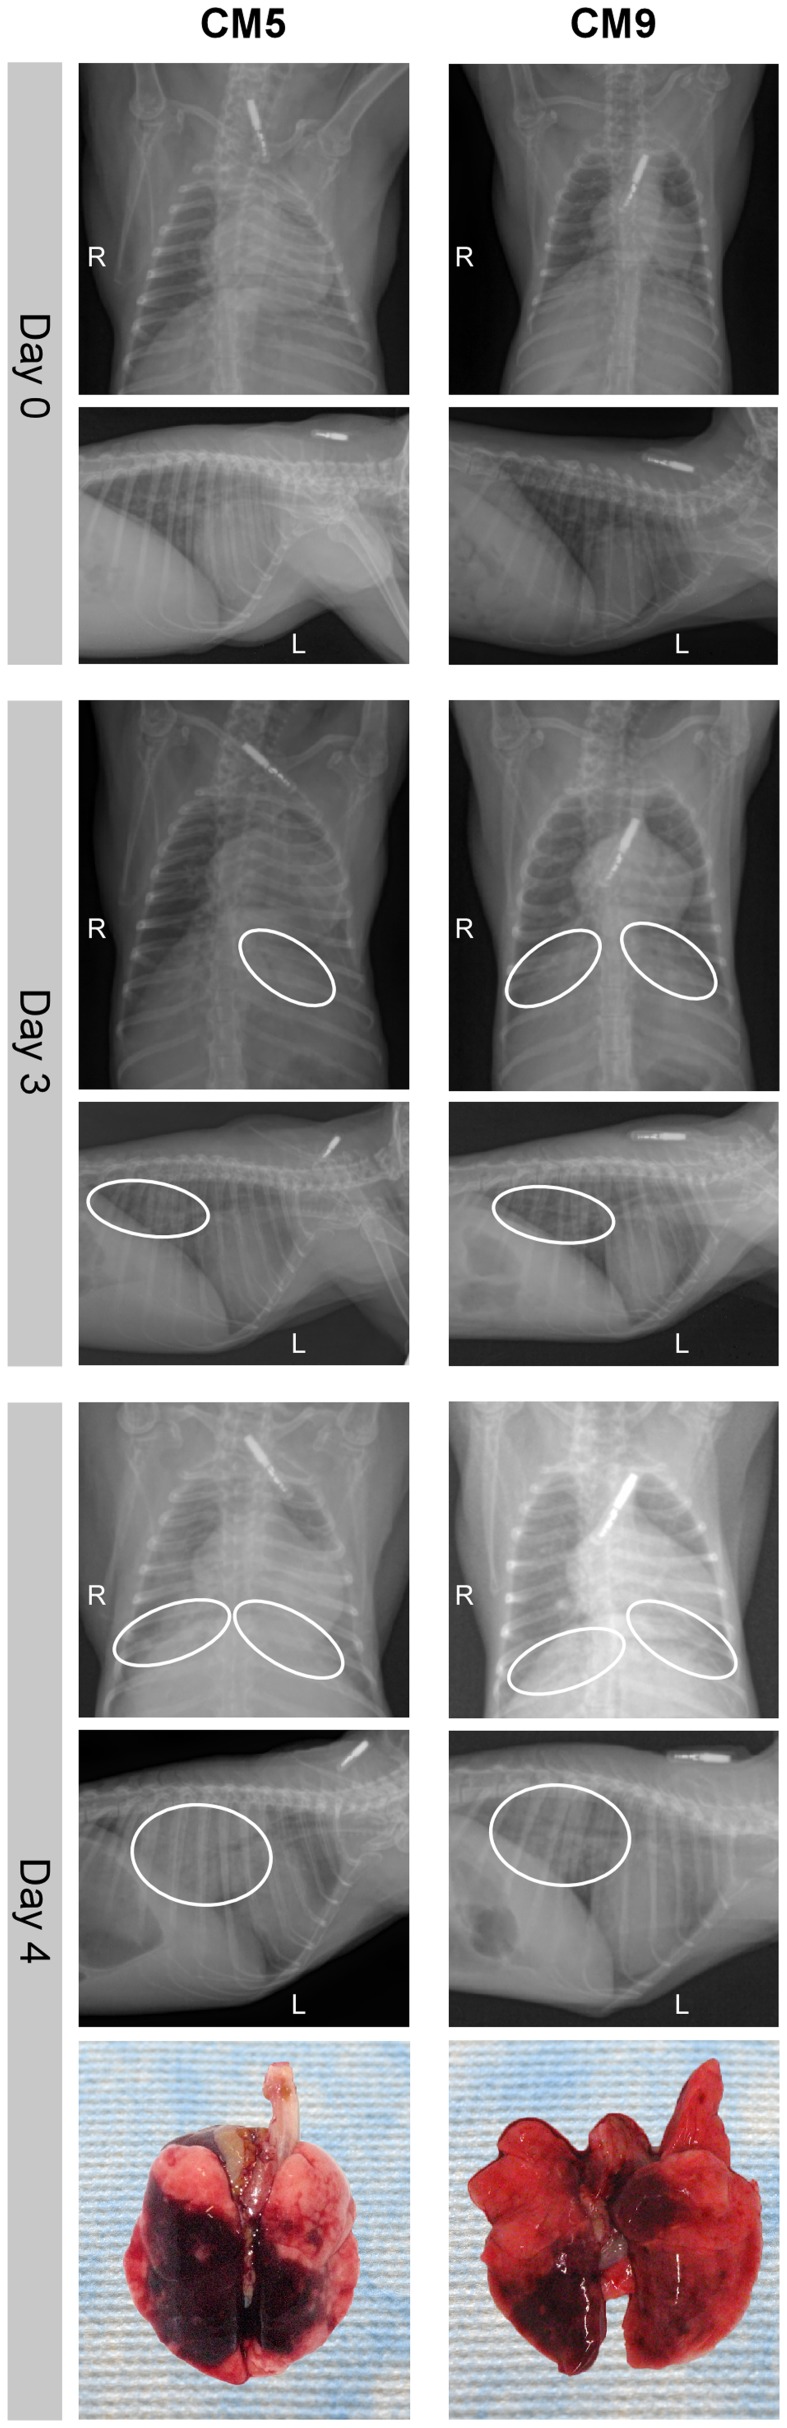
Radiographic alterations and lung pathology. Dorsal-ventral and lateral thoracic x-rays from of common marmosets (CM) imaged prior to MERS-CoV inoculation (day 0) and on days 3 and 4 post-inoculation. Areas of interstitial infiltration, indicative of pneumonia, are highlighted (circle). Positional indicators are included (R – right, L – left). Gross pathology of the lungs from CM5 and CM9 necropsied on 4 dpi are shown below indicating extensive gross lesions. dpi are shown below indicating extensive gross lesions.
